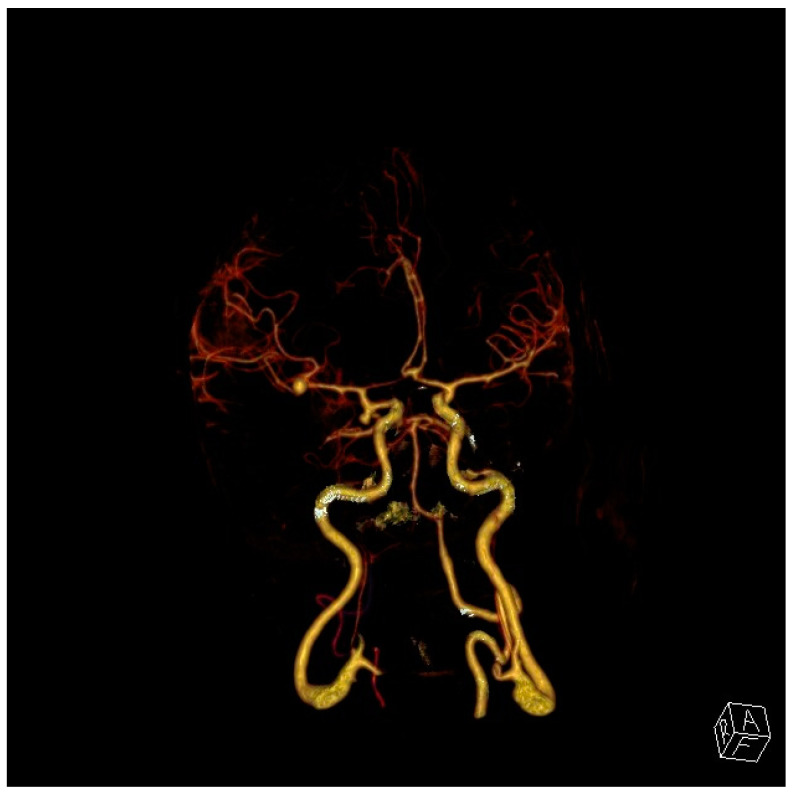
CT angiogram of the same patient shows two right-sided saccular aneurysms (left side of figure), the larger at the right MCA bifurcation and the second arising from the right ICA (up to 20% of aSAH patients have more than one aneurysm). Given that the MCA aneurysm was larger and associated with thicker Sylvian and insular clots, we could be confident it was the source of the bleeding, but both aneurysms were repaired with microsurgical clipping on the day of admission.
